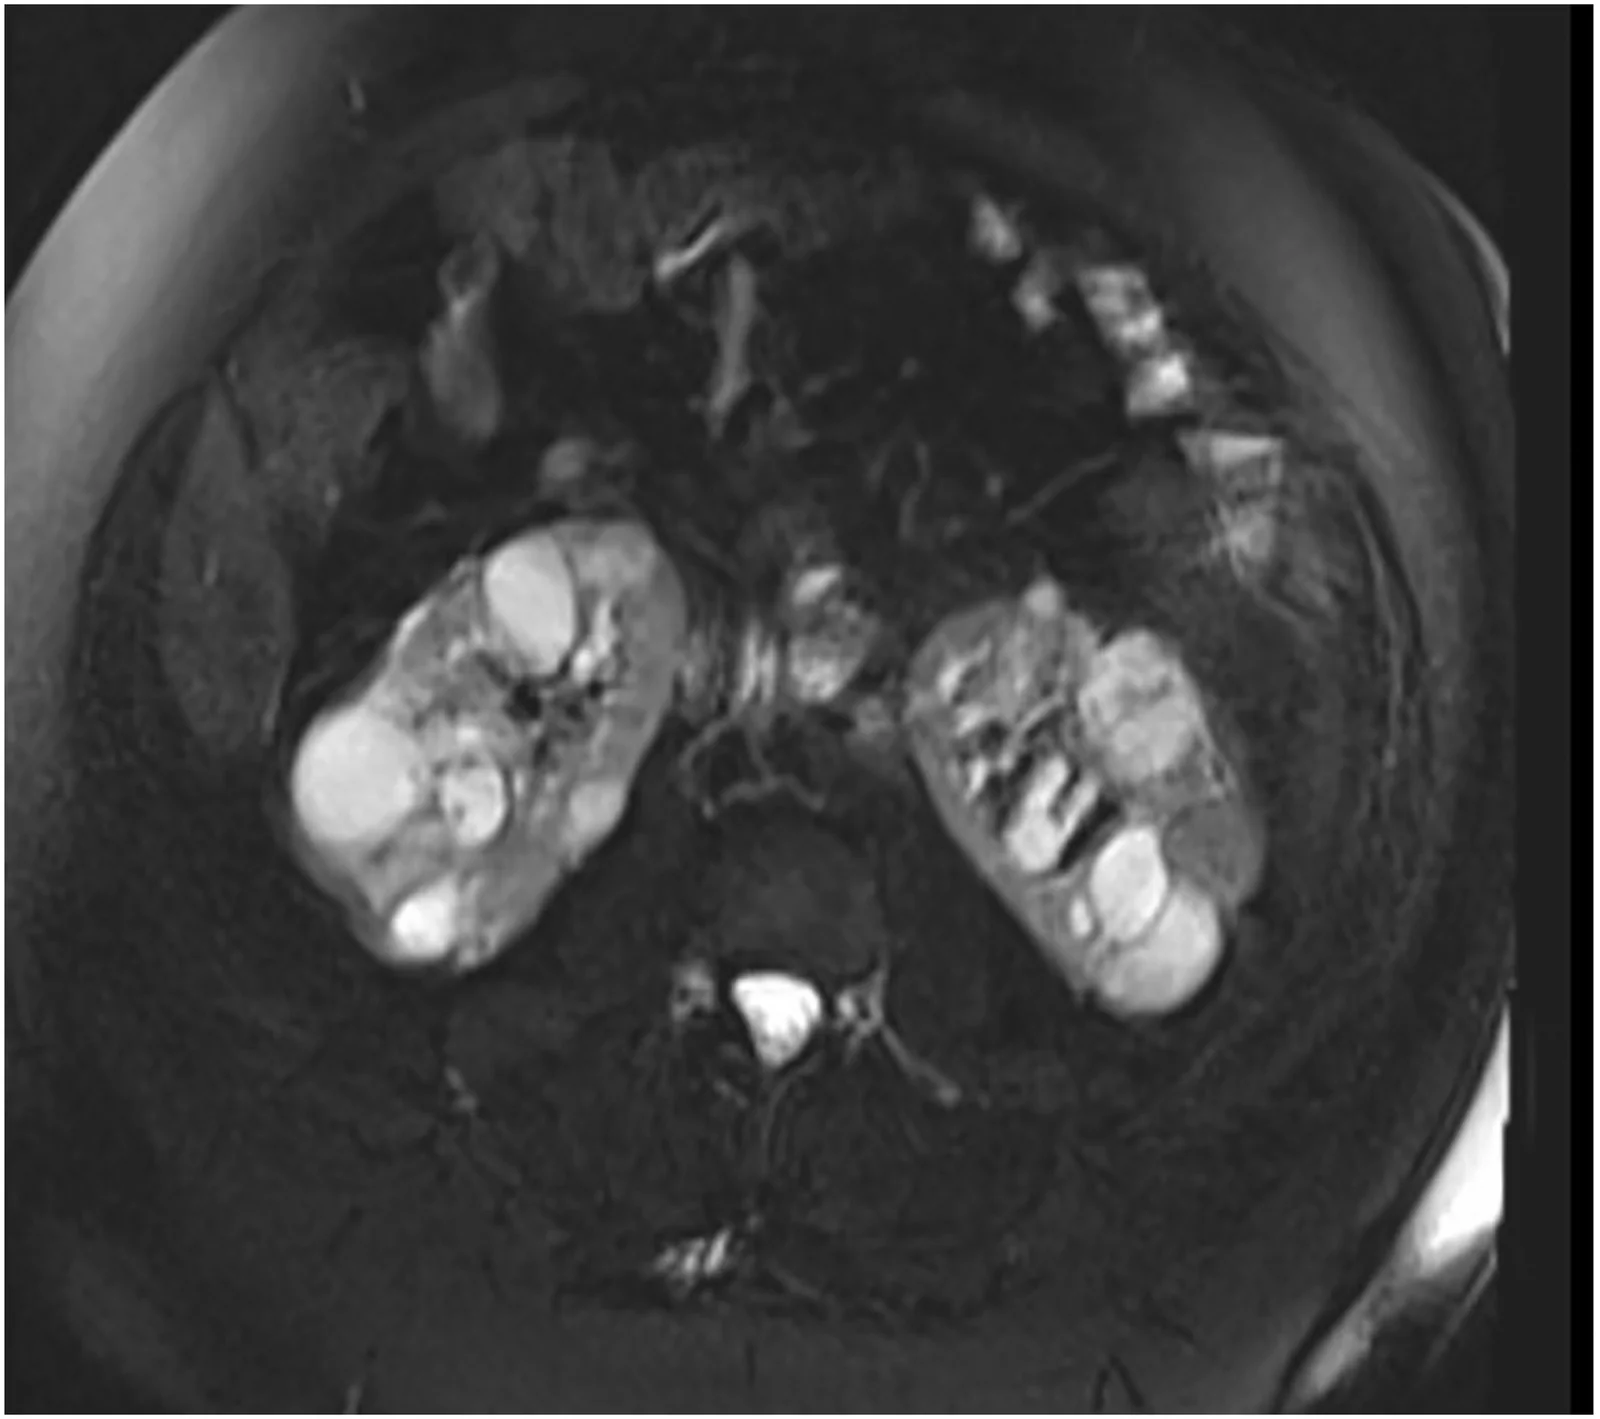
Case 3. Coronal MRI showing a 2.4cm solid enhancing mass in the interpolar region of the left kidney
